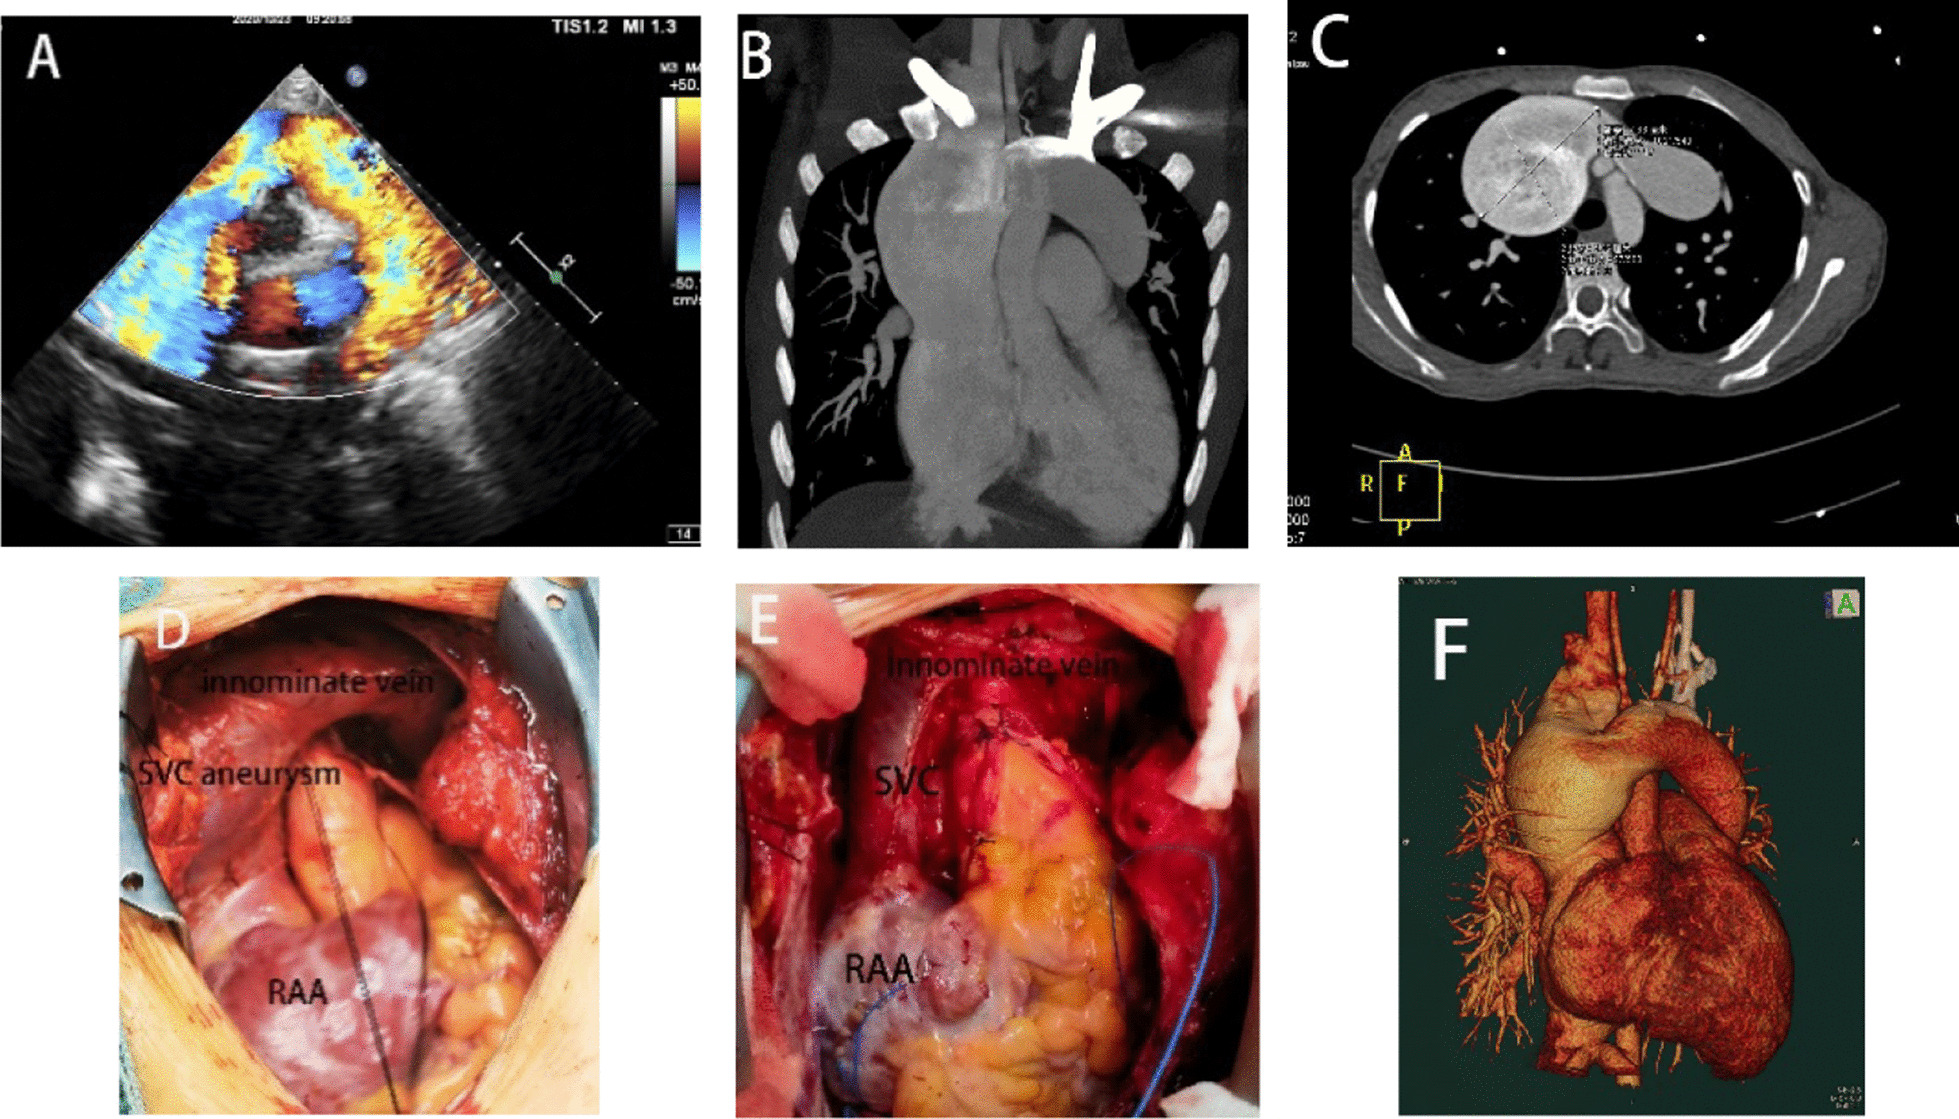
a–b; f Preoperative transthoracic echocardiography and CT showed supracardiac TAPVC with a giant venous arch. C CT showed the maximum diameter of the SVC. d–e Pre and postoperative view of SVC
